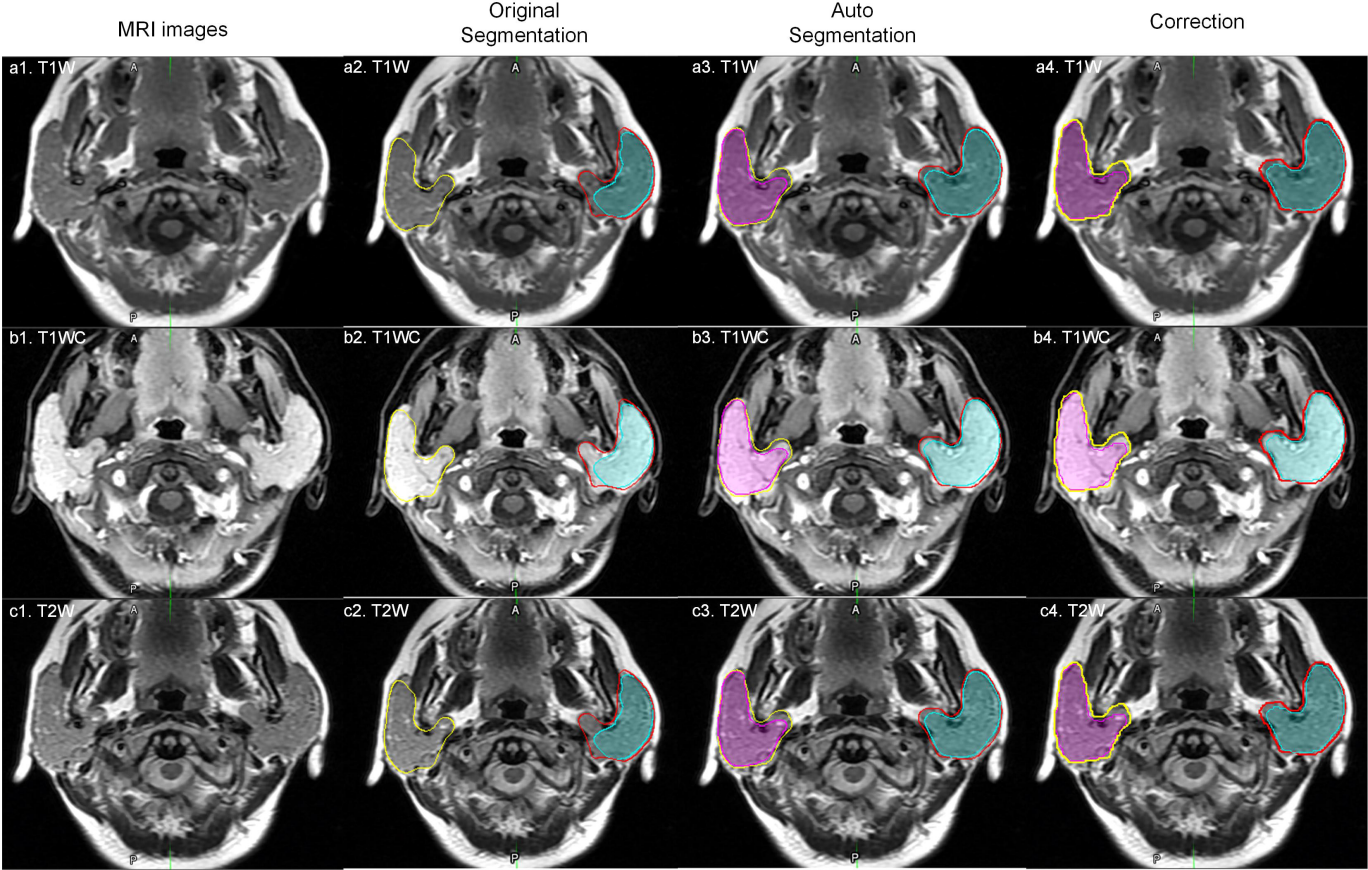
An outlier example. The yellow and red lines represent the right and left parotid. The pink and cyan colored filling represents the right and left tumor. a1, b1 and c1 represent one slice of MR images; a2, b2 and c2 represent original manual segmentation. The right tumor was not delineated correctly; a3, b3 and c3 represent the results of autosegmentation; a4, b4 and c4 represent the corrected segmentation by manual delineation by physicians.
